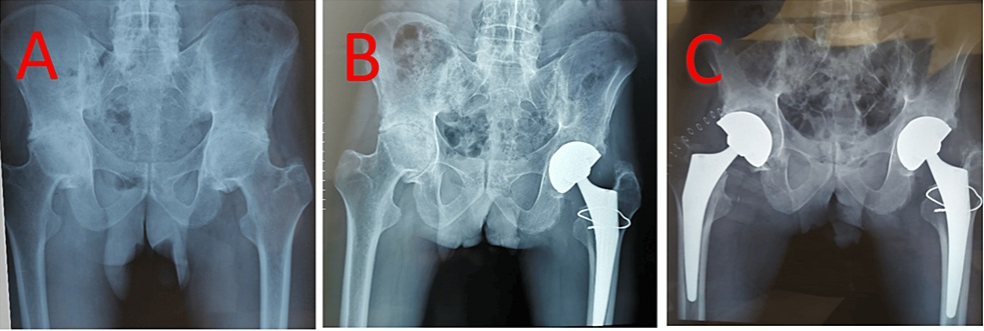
Radiographic assessment of case 2 A: Initial radiograph. B: Follow-up radiograph after placement of the left prosthesis. C: Follow-up radiograph after fitting the right prosthesis.
